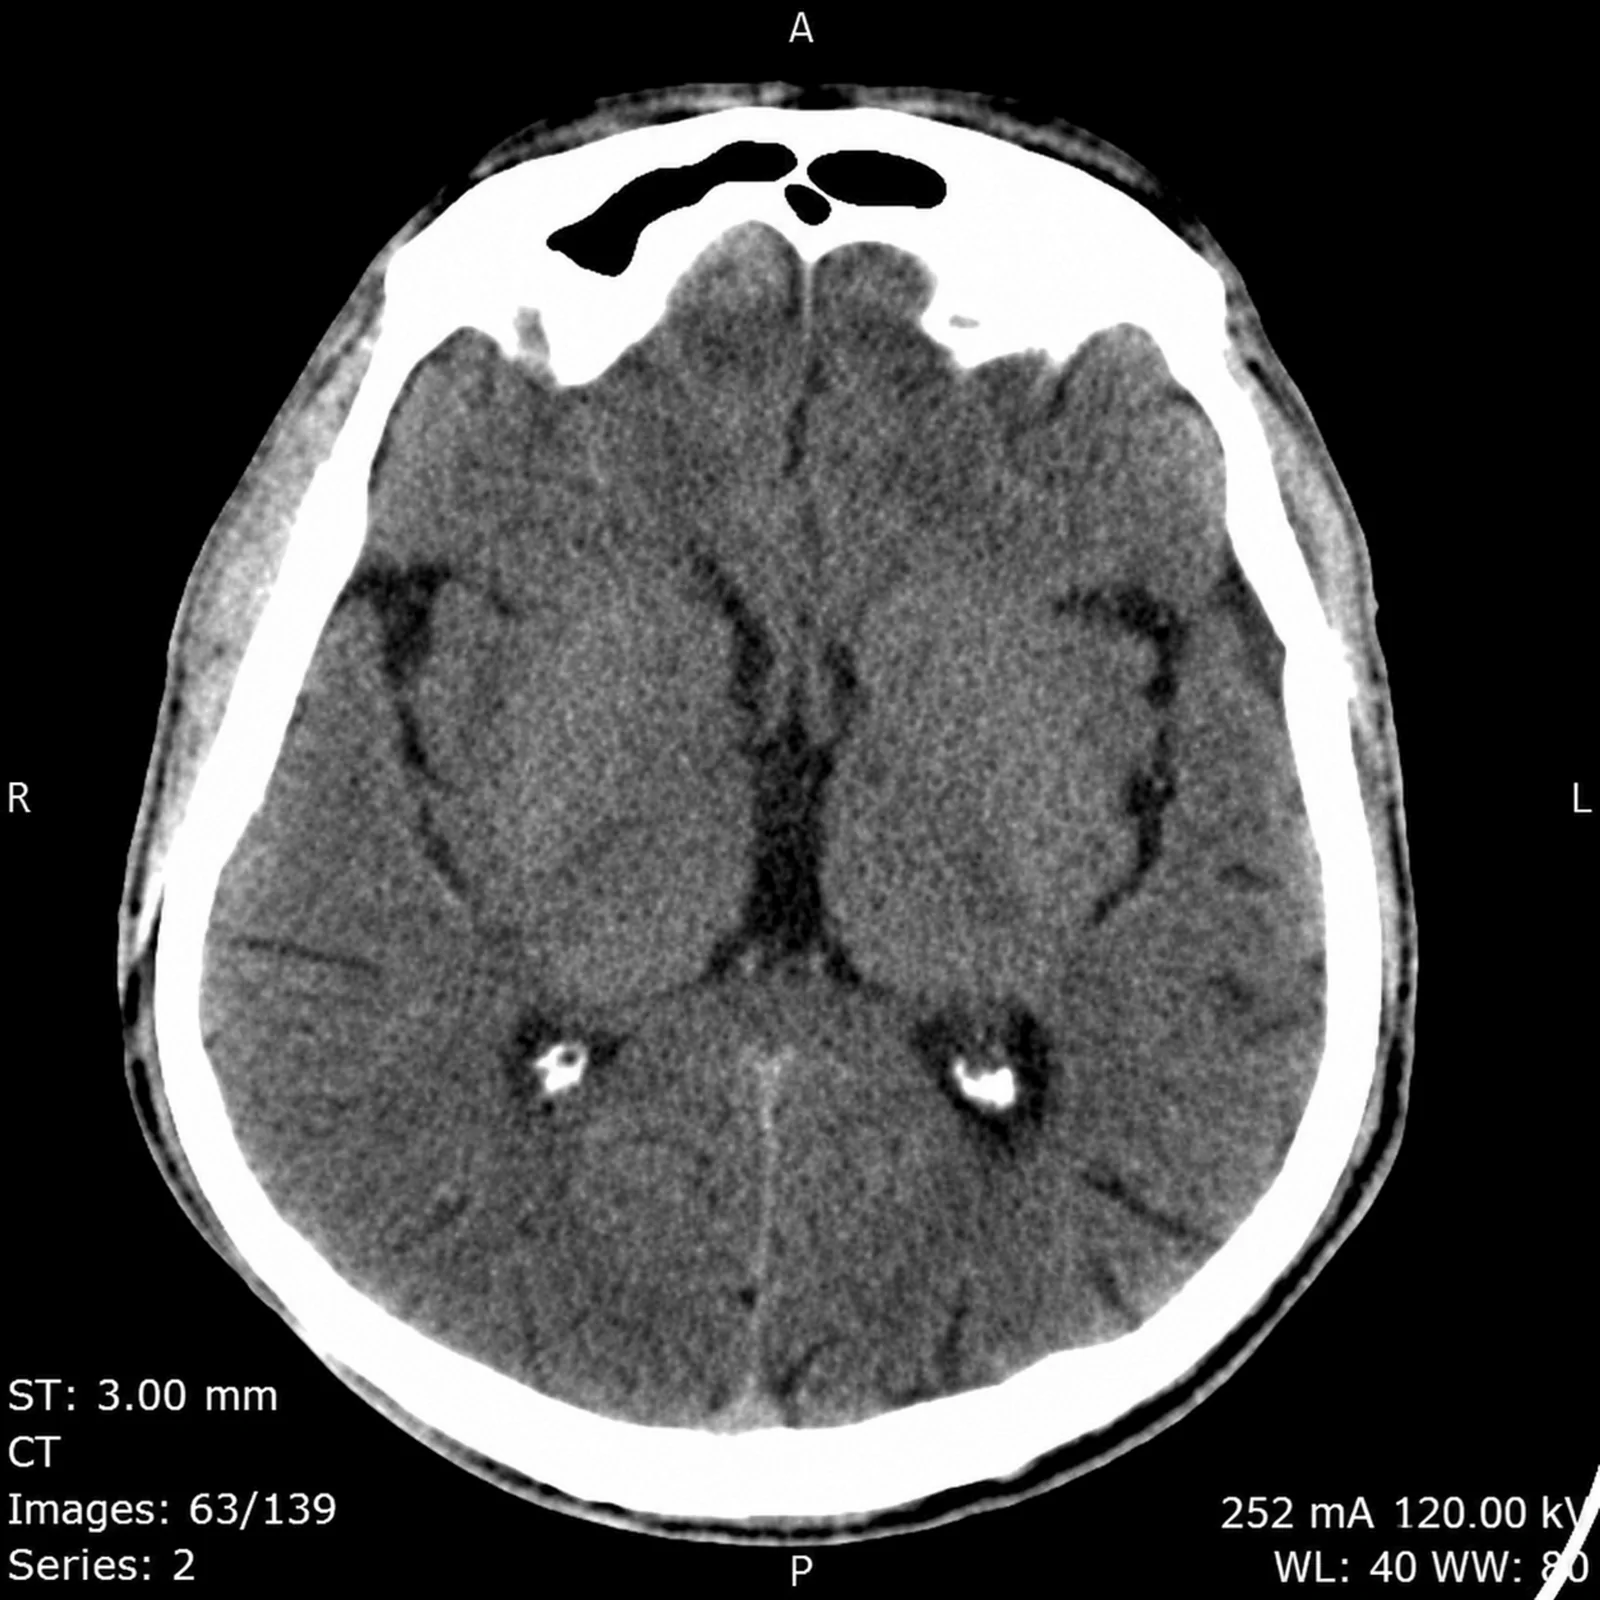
Axial non-contrast computed tomography image of the head. Representative axial non-contrast computed tomography image showing no acute intracranial hemorrhage or significant mass effect.
